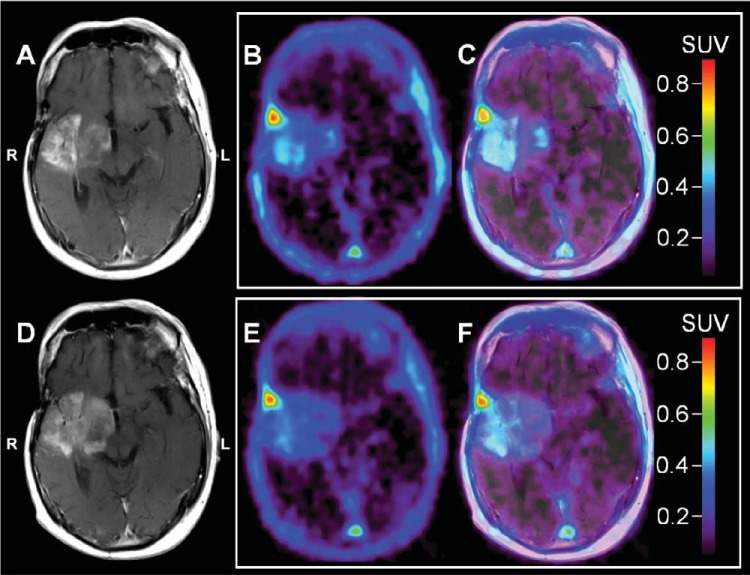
Patient ML-10 #2. Representative baseline (BL; A–C) and early-therapy assessment (ETA; D–F) contrast enhanced (CE) MRI, [^18^F]ML-10 standard uptake value (SUV), and PET/CE-MRI fusion images for a 48 years old male with a recurrent GBM diagnosis. All images are shown coregistered to the patient's BL CE-MRI. BL CE-MRI (A) shows an infiltrative mass involving portions of the right frontal and temporal lobes, with the greatest involvement in the right insular cortex anterior temporal lobe and right basal ganglia. ETA CE-MRI (D) shows progression of disease compared with BL as evidenced by increased infiltration, in particular, of the right insular region into the right basal ganglia with some increased mass effect on the third ventricle, which is displaced slightly toward the right. There is also an increase in the extent of associated enhancement and extension into the right cerebral peduncle. On PET, BL [^18^F]ML-10 SUV image (B) shows mild therapy-naive uptake (BL SUVmax = 0.54) located at the tumor site on PET/CE-MRI fusion (C). The ETA [^18^F]ML-10 SUV image (E) shows little change in [^18^F]ML-10 uptake (ETA SUV_max_ = 0.48) at the tumor site (F) consistent with minimal changes in GBM apoptosis rate compared with BL.
